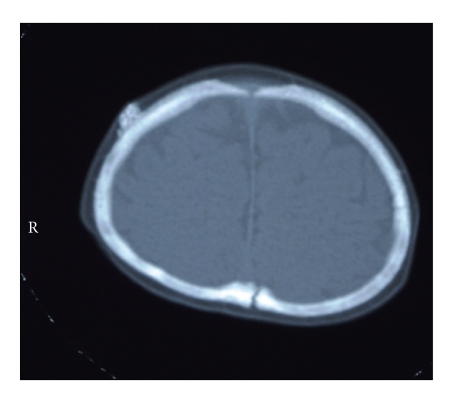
Skull CT image of patient 2 taken at 7 months of age. Calcification at right parietal region is evident.
